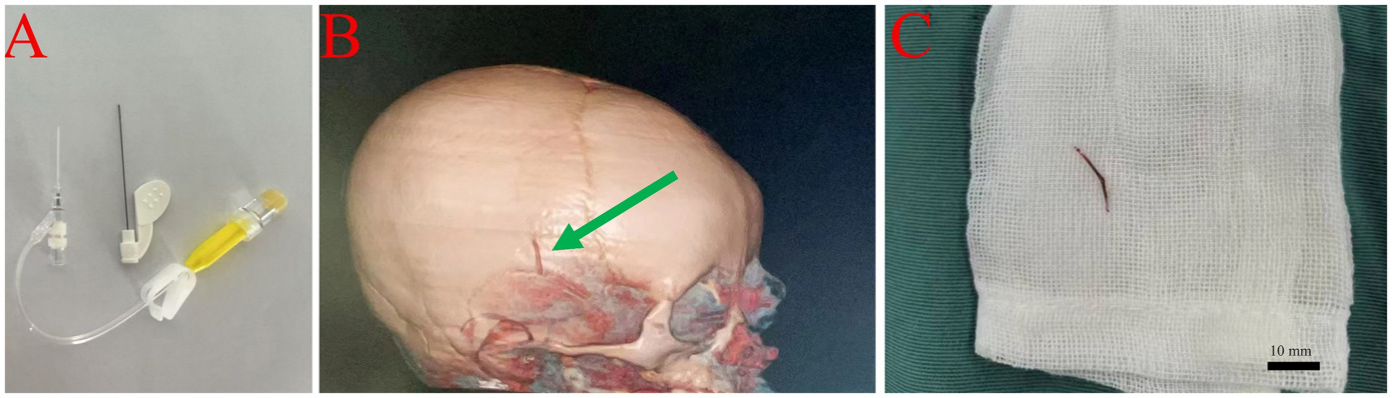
Removal of subcutaneous catheter fragment in a 1-year-3-month-old male infant. (A) Photograph of a newly inserted peripheral catheter at the affected site, prepared for surgical intervention. (B) Three-dimensional computed tomography (CT) angiography of the head, showing a 1.5 cm fractured catheter fragment within the right superficial temporal vein, as indicated by the green arrow. (C) Intraoperative photograph of the retrieved catheter fragment, measuring approximately 1.5 cm, with a scale bar, following surgical excision under general anesthesia.
